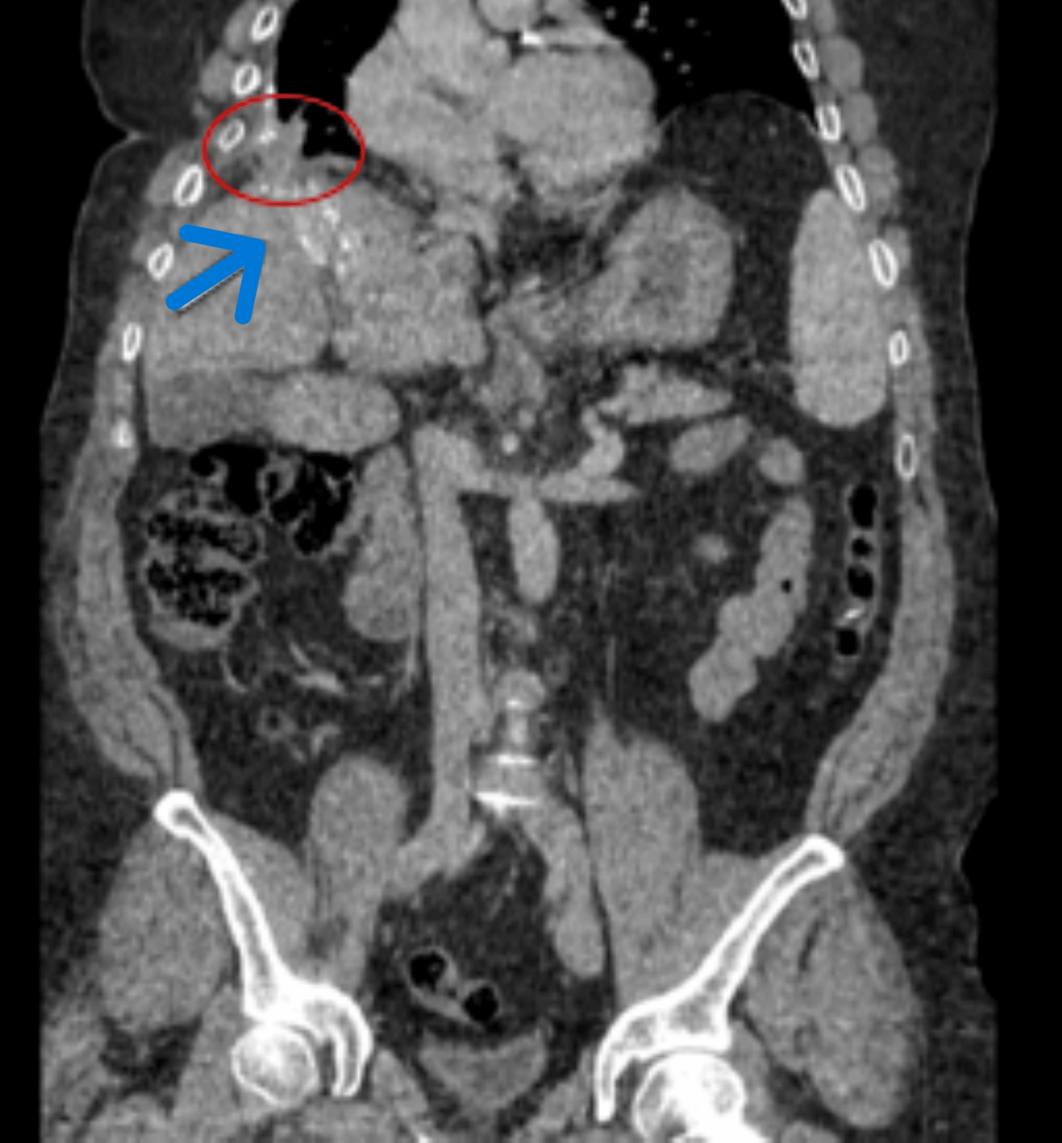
Computed tomography scan of control after six years demonstrating residual hepatic calcifications (blue arrow) and fibrosis resulting from hepato-pleural fistula (within the red circle) and absence of recurrence.
